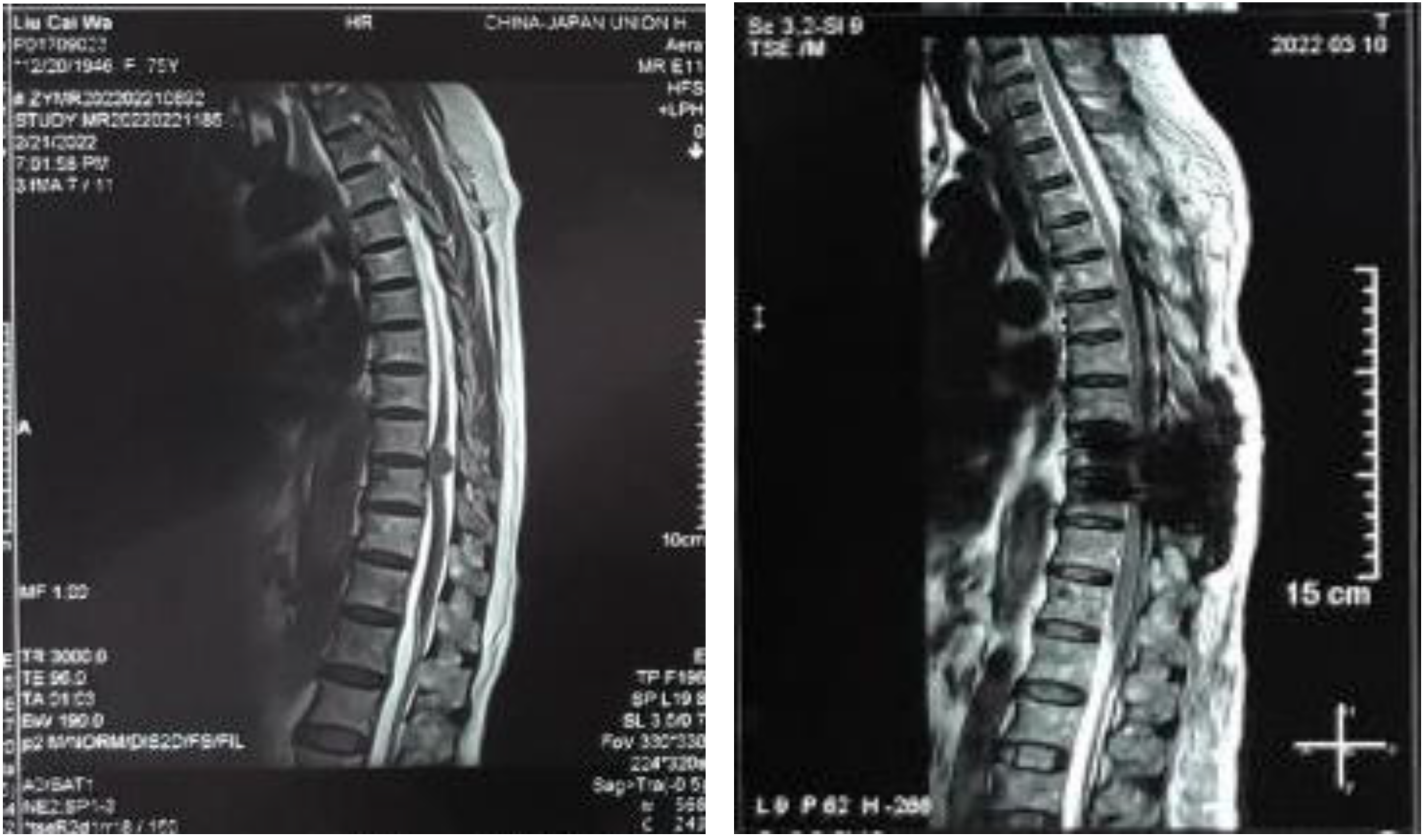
Thoracic lumbar spine MRI scan findings. The figure shows an intraspinal space-occupying lesion at the level of the patient’s T9-T10 intervertebral disc, which was confirmed by imaging as the source of the spinal lesion. The image was a preoperative finding that showed the exact location of the lesion and provided an important basis for the subsequent surgical plan.
